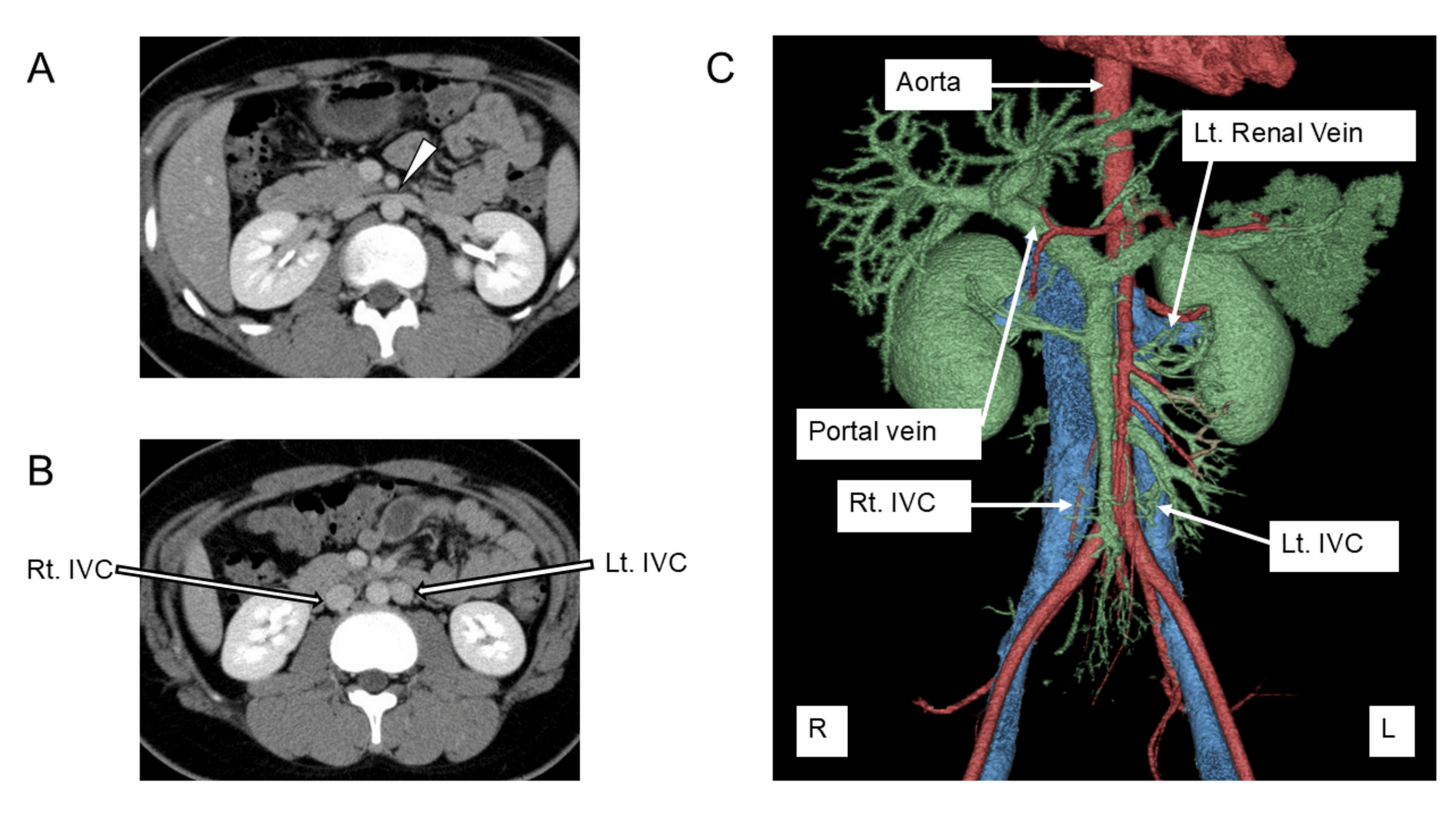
Axial (A, B) and three-dimensional (C) reconstructed contrast-enhanced CT images demonstrating double inferior vena cava. The right and left common iliac veins independently drain into their respective IVC. Notably, compression of a vessel (arrowhead) is observed at the confluence of the left IVC and the left renal vein, anterior to the abdominal aorta. IVC: Inferior vena cava
